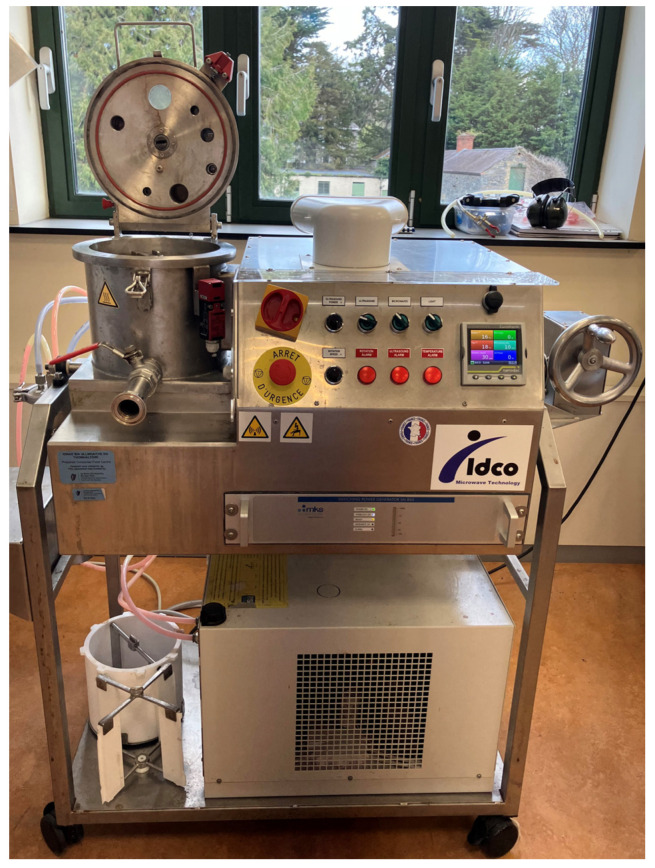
E200 Ultrasound–Microwave extractor (IDCO, Marseille, France).
